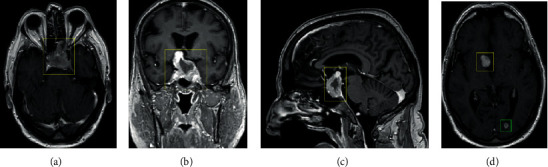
Radiographic imaging of tumor progression preoperatively. T1-weighted sequence magnetic resonance imaging with contrast presented in axial, coronal, sagittal, and axial planes, respectively. Imaging demonstrates growth of the previously described mass with further encasement of the left cavernous internal carotid artery (a) with superior extension (b) with further effacement of the third ventricle (c). A subcentimeter-enhancing, spherical, paraventricular, left occipital lesion is apparent (d). The sellar mass now measures 5.7 x 3.8 x 4.4 cm with a total estimated volume of 22.7 mL. Yellow boxes highlight the tumor of interest. Green box highlights an occipital lesion.
